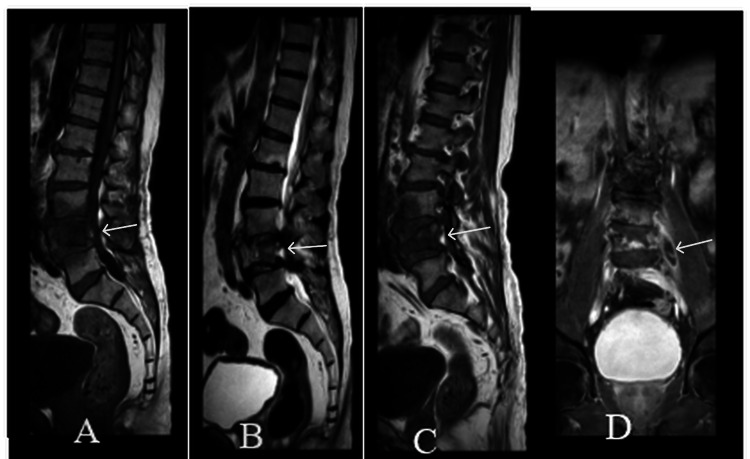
MRI of the lumbosacral spine (Case 2) A) Sagittal T1-weighted image: altered marrow signal at L4 and L5 vertebral endplates (arrow). (B) Sagittal T2-weighted image: hyperintense inflammatory changes with disc involvement at L4–L5 (arrow). (C) Coronal image: left psoas muscle collection consistent with abscess formation (arrow). (D) Axial image: prevertebral soft tissue extension adjacent to the involved vertebral bodies (arrow).
